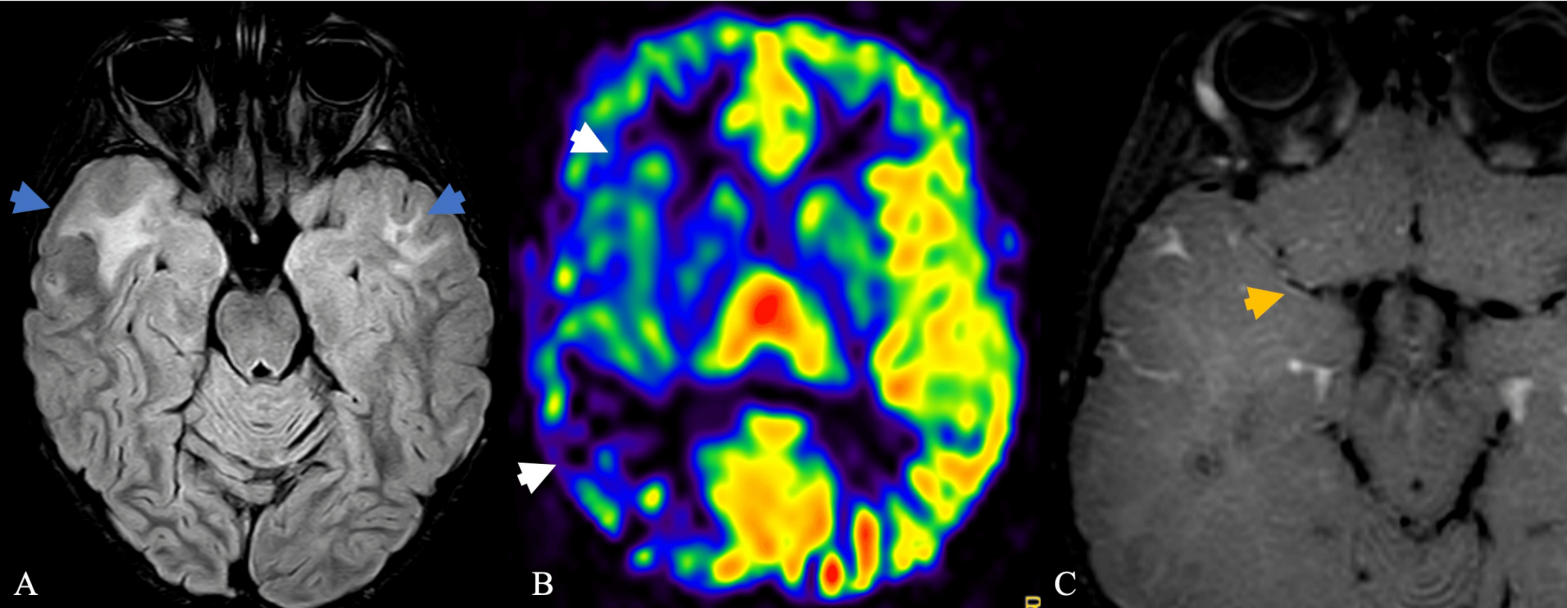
Axial MRI brain was acquired during hospitalization, approximately five years after the last brain radiation (A) FLAIR sequence shows anterior temporal lobe white matter hyperintensity (blue arrow). (B) Arterial spin-labeled imaging of the brain at the level of the caudate and putamen shows decreased blood flow within the right MCA territory (white arrow). (C) Post-contrast black blood imaging through the level of the circle of Willis shows mild enhancement circumferentially around the area of the narrowed M1 segment of the right MCA (yellow arrow). FLAIR: fluid-attenuated inversion recovery; MCA: middle cerebral artery
